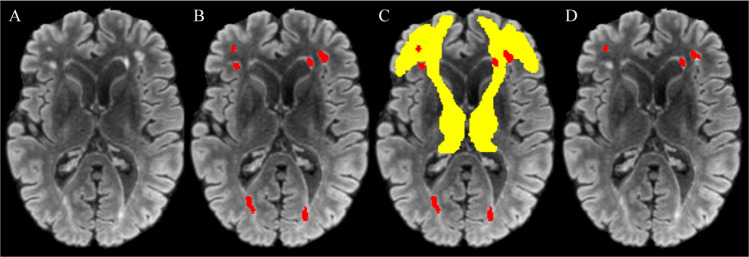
Steps to derive tract specific WML volumes. MS-GAN was applied to A each participants FLAIR scan to derive B binary whole brain lesion masks (red). C The intersection of tract masks computed from the DWI template (non-linearly registered to subject space; ATR shown in yellow) and the whole brain lesion masks was calculated to derive D the final tract specific binary lesion masks for statistical analysis.
